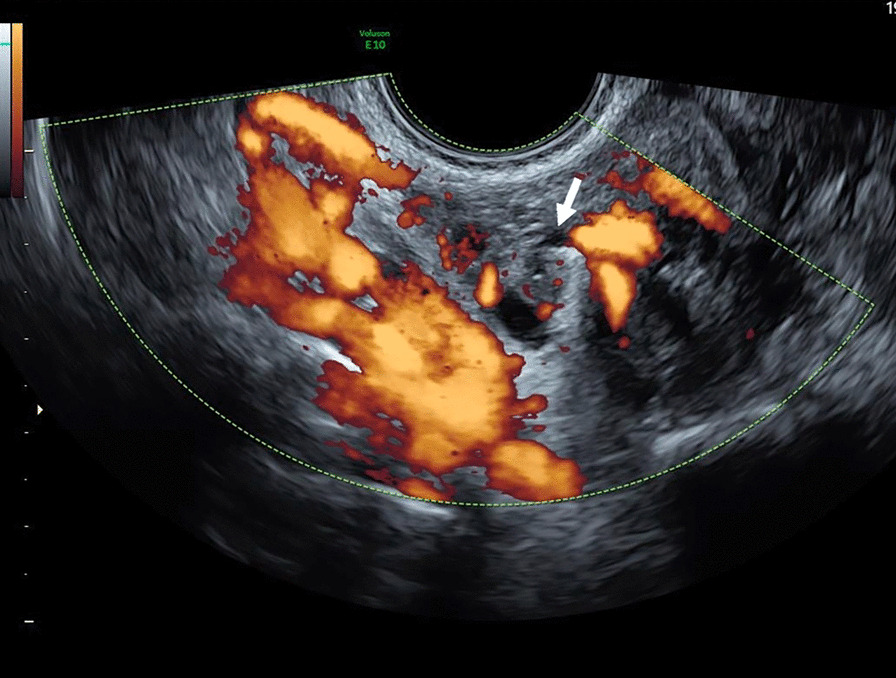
Color Doppler transvaginal ultrasonography: twisted ovarian pedicle (arrow)
